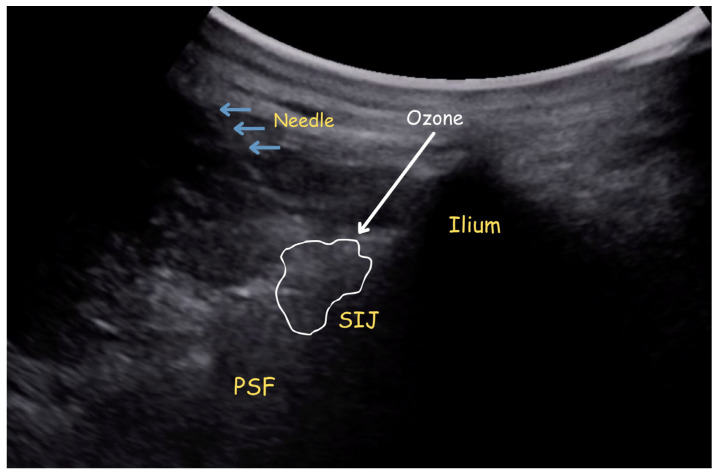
Ultrasound image demonstrating the needle trajectory, ozone distribution, and relevant anatomical structures during sacroiliac joint injection. PSF: posterior sacral foramen, SIJ: sacroiliac joint.
